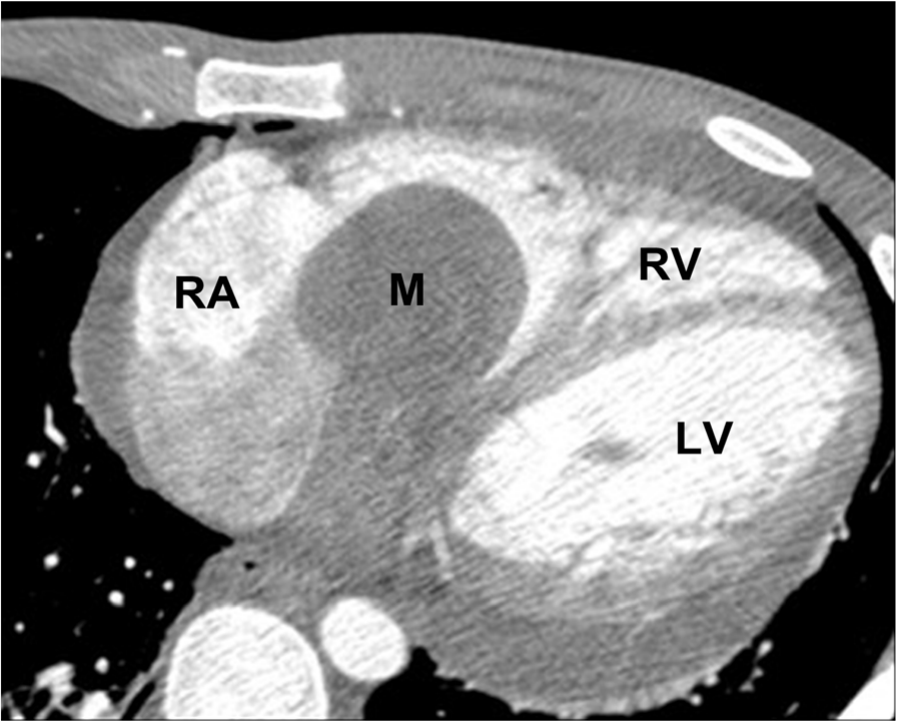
Axial contrast-enhanced CT shows a pedunculated myxoma in the right atrium, which extends through the tricuspid valve into the right ventricle. *RA* right atrium, *M* myxoma, *RV* right ventricle, *LV* left ventricle
